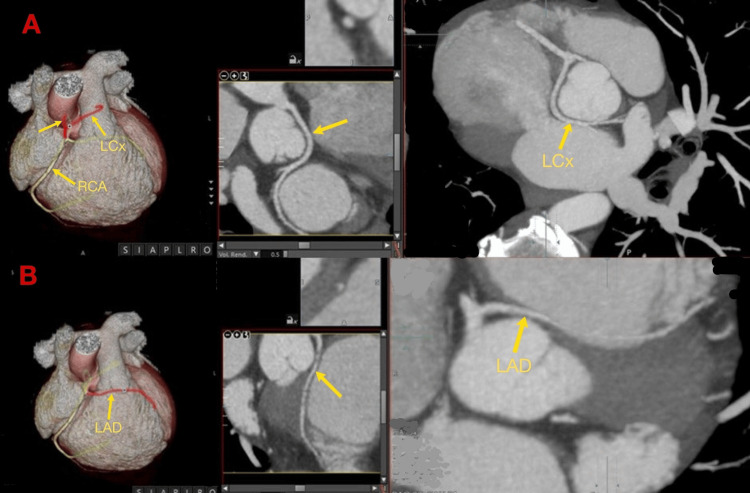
MDCT: demonstrating the origin of the coronary arteries arising from the right coronary sinus-the retroaortic course of LCx (A) and the interarterial course of the LAD (B). MDCT: multidetector CT; LCx: left circumflex; LAD: left anterior descending; RCA: right coronary artery
